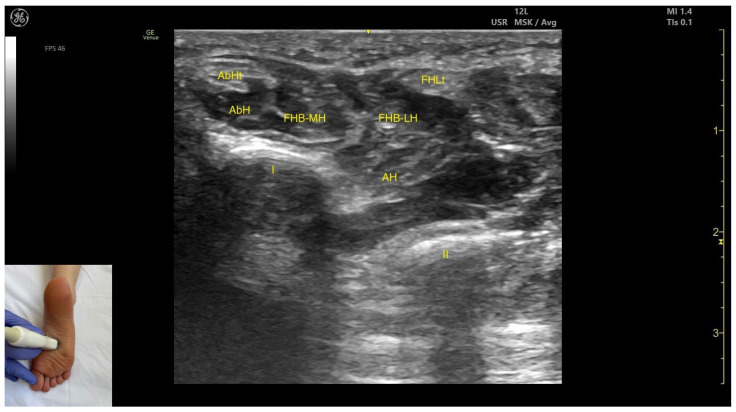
Ultrasound anatomy of the flexor hallucis brevis: AbH—abductor hallucis; AbHt—abductor hallucis tendon; FHB-MH—flexor hallucis brevis medial head; FHB-LH—flexor hallucis brevis—lateral head; FHLt—flexor hallucis longus tendon; I—first metatarsophalangeal joint; II—second metatarsal joint, AH—adductor hallucis.
